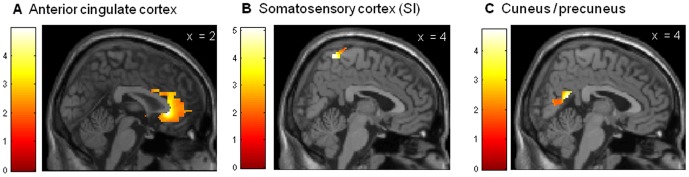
Neural activation within regions-of-interest during early acquisition {CS^+^>CS^−^}. Neural activation during anticipatory conditioned responses in the early acquisition phase assessed within ROIs with a one-sample t-test on the contrast CS^+^>CS^−^ with increase in CS^+^ unpleasantness as covariate of interest. During early acquisition, presentation of the CS^+^ led to significantly greater anticipatory activation in the anterior cingulate cortex (Fig. 3A), somatosensory cortex (Fig. 3B), and the cuneus/precuneus (Fig. 3C) when compared to the CS^−^. All p_FWE_<.05, for more details, see Table 2. Activations were overlaid on a structural T_1−_weighted MRI used for spatial normalization and thresholded at p<.01 for visualization purposes using anatomical templates.
